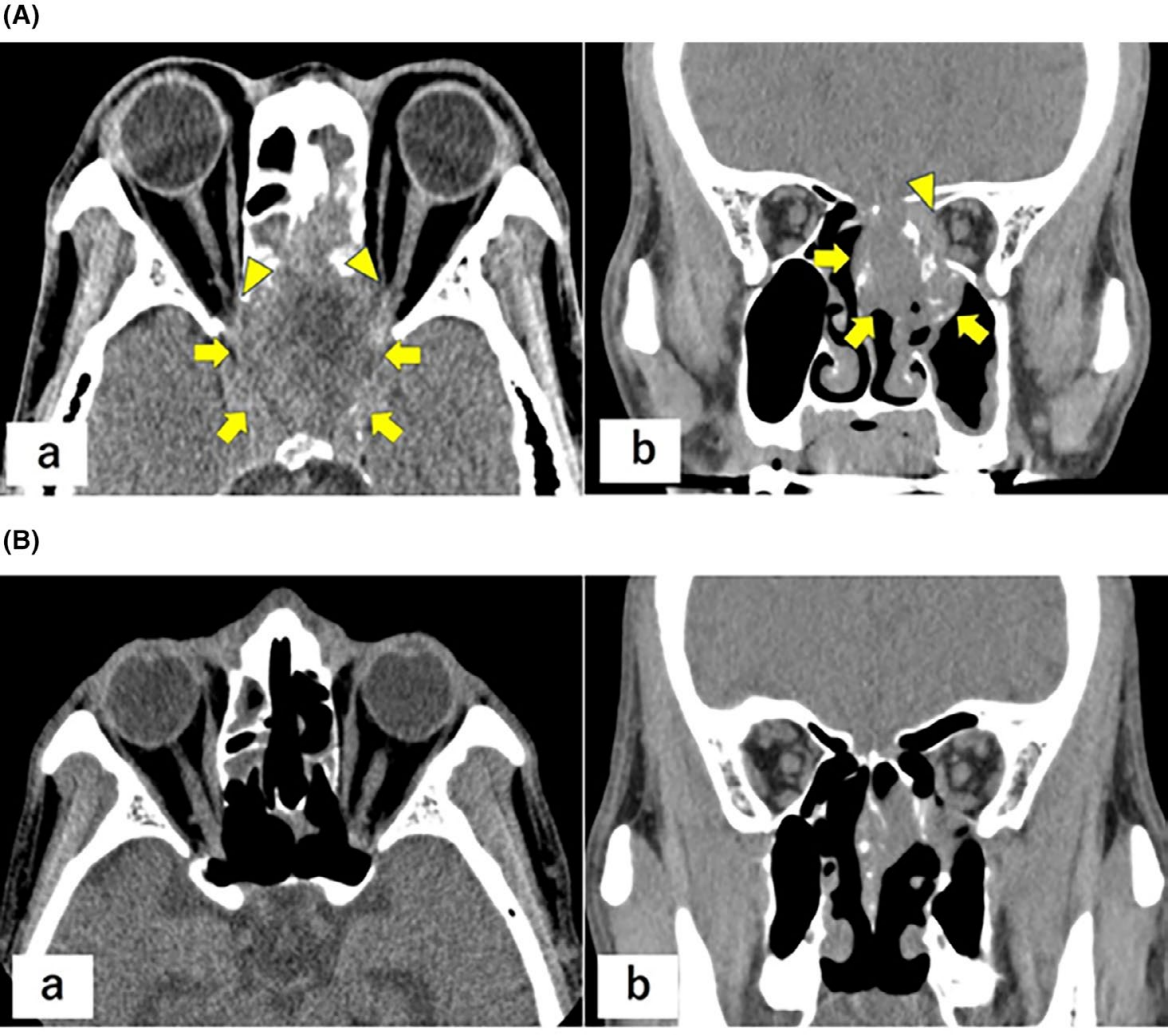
Non‐contrast head computed tomography images showing interval enlargement of the sphenoid sinus tumor with involvement of both optic canals, consistent with optic pathway compromise. (a) Axial image. (b) Coronal image. Arrows: tumor; arrowheads: optic canals. Non‐contrast head computed tomography images during follow‐up demonstrating interval shrinkage of the sphenoid sinus lesion after radiotherapy and immunotherapy, consistent with a partial response. (a) Axial image. (b) Coronal image. [Correction added on 20 March 2026, after first online publication: Figure 3 and its caption have been corrected.]
